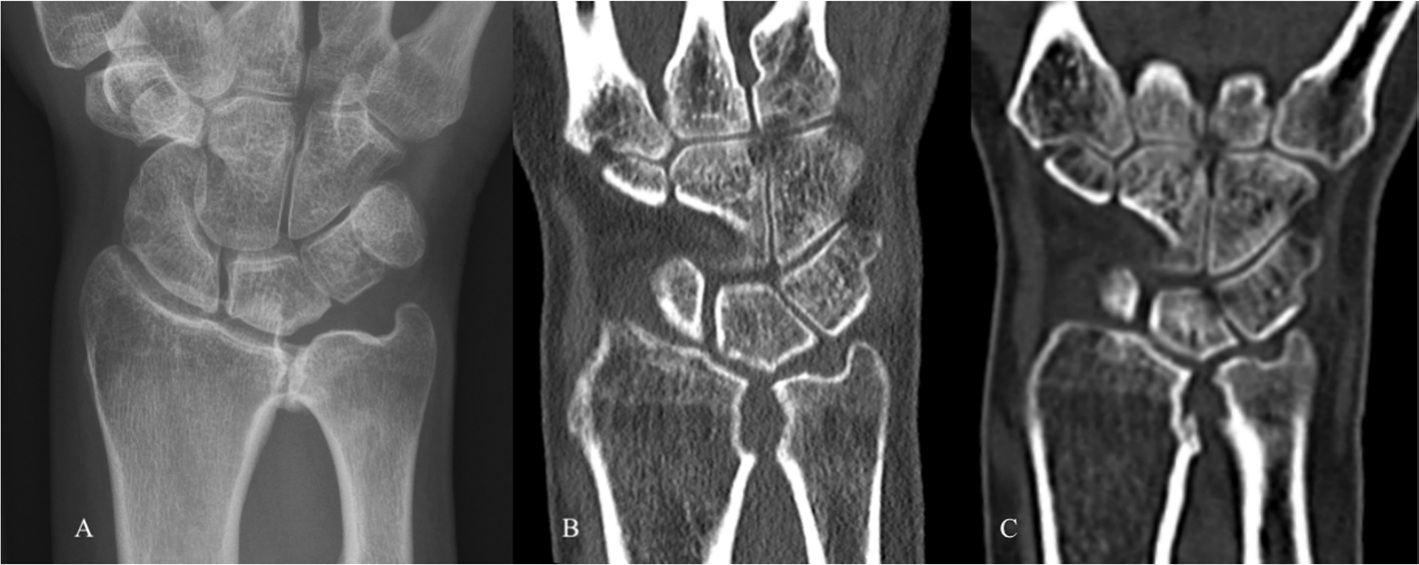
PA radiographs in case 3 with osteoarthritis in the DRUJ (A). Computer tomography (CT) at 6 months follow-up showing a wide distance in the joint (B), which is unchanged 5 years postoperatively (C)
